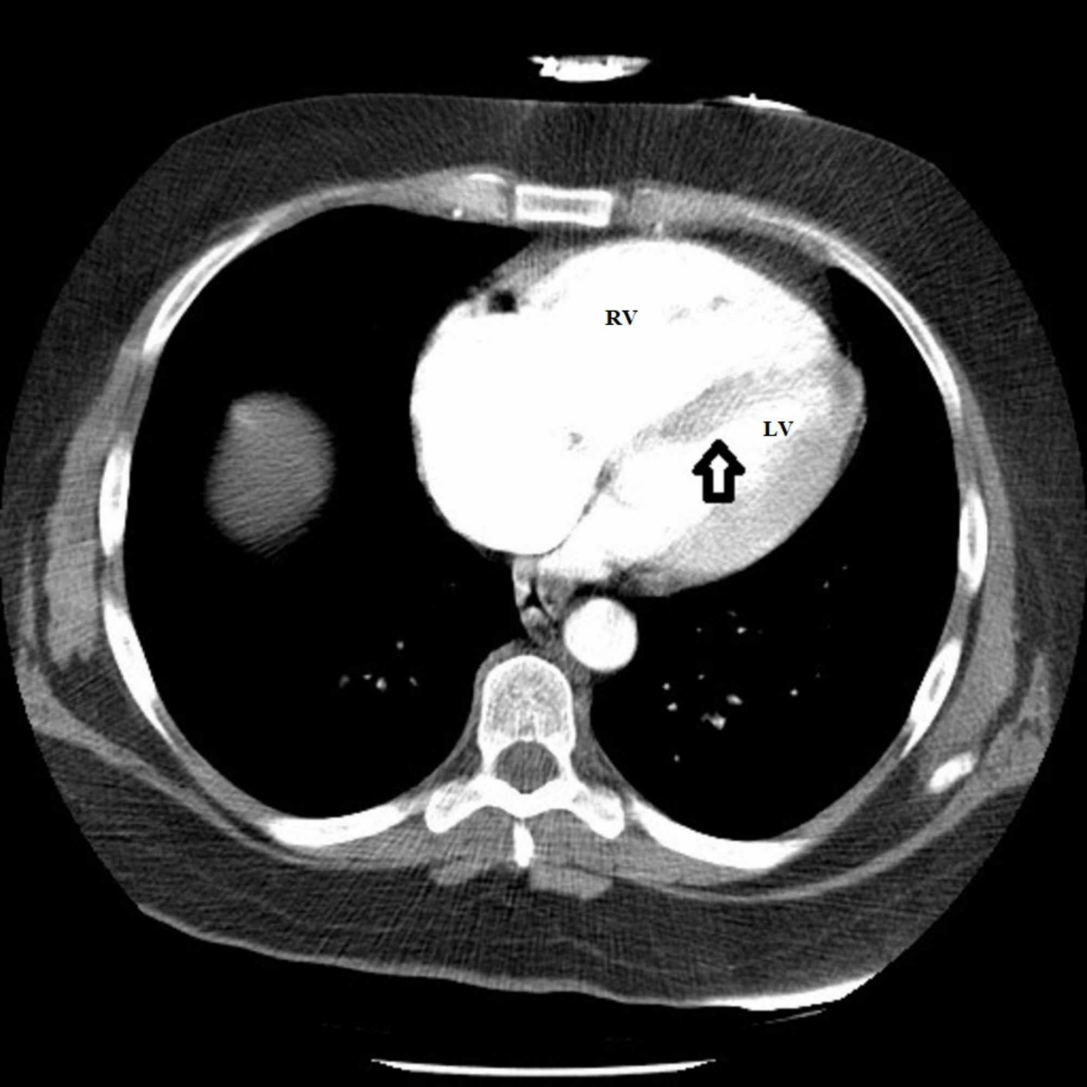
CT pulmonary angiogram showing flattening of the interventricular septum (arrow) with an increase in the size of the right ventricle (RV) and reduced left ventricular (LV) size
